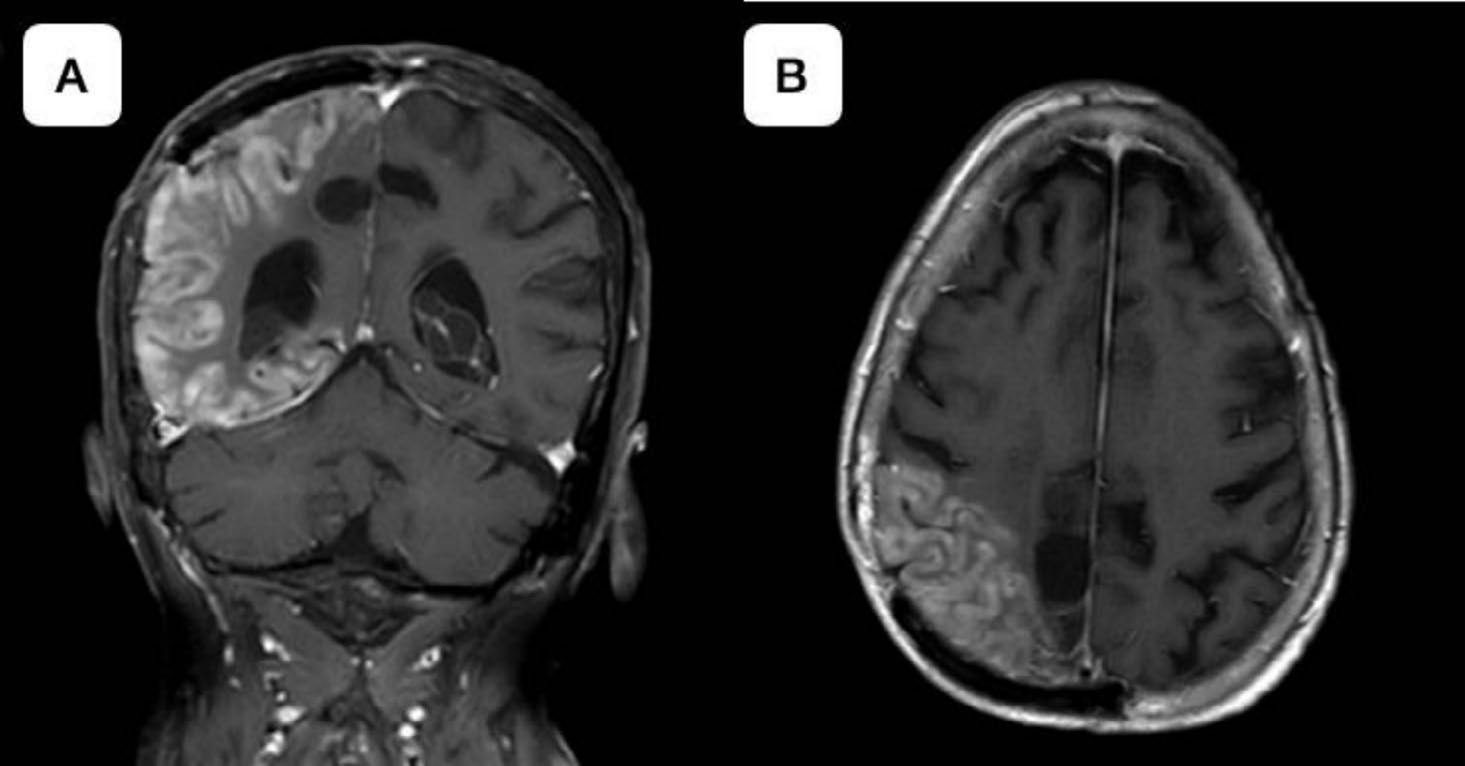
Contrasted MRI Brain. (A) Coronal view and (B) Axial view showing right parietal, temporal, occipital gyral enhancement with evidence of cortical swelling and mild diffusion restriction in DWI sequences in previously irradiated areas.
